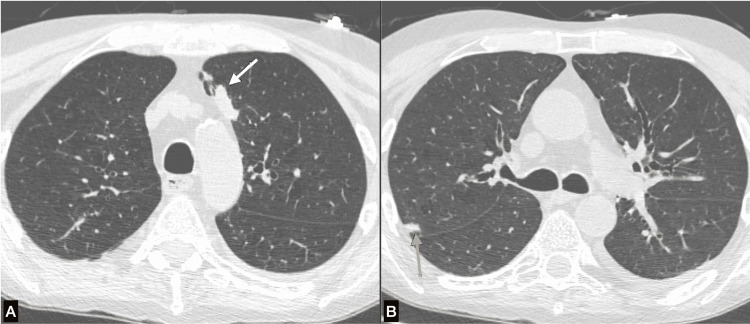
Axial CT image (A) shows a lobulated solid pulmonary nodule in the anterior segment of the left upper lobe adjacent to the mediastinal pleura (straight white arrow). Axial CT image (B) shows a small, slightly irregular-shaped solid pulmonary nodule in the posterior segment of the right upper lobe adjacent to the costal pleura (straight yellow arrow).
